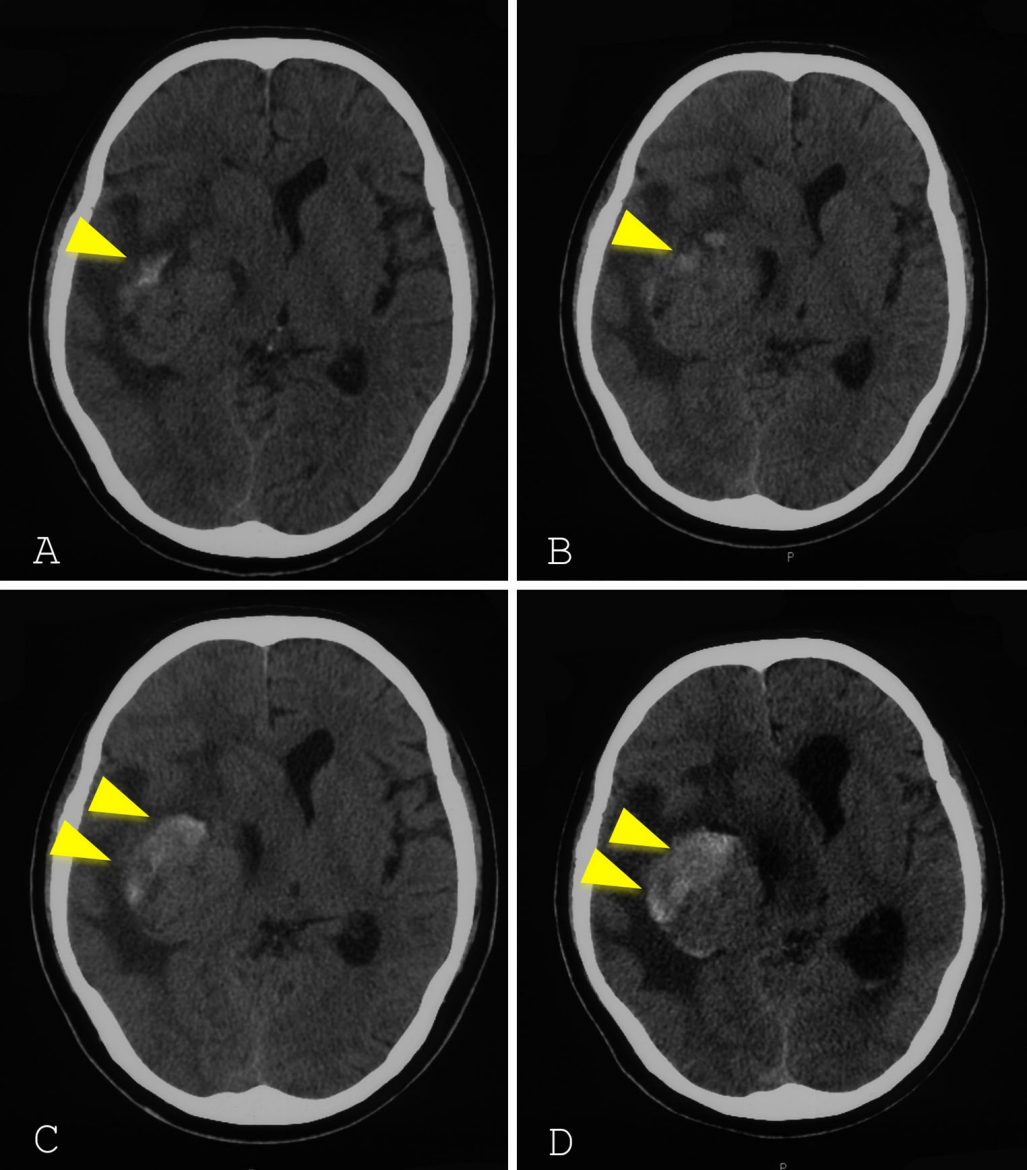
Computed tomography scans obtained on December 26, 2008 (a), December 31, 2008 (b), January 3, 2009 (c), and January 7, 2009 (d). A high-density area can be seen in the tumor that gradually expands (*arrow head*). This indicates repeated intratumoral hemorrhage. The perilesional brain edema and displacement of the midline structures deteriorated
